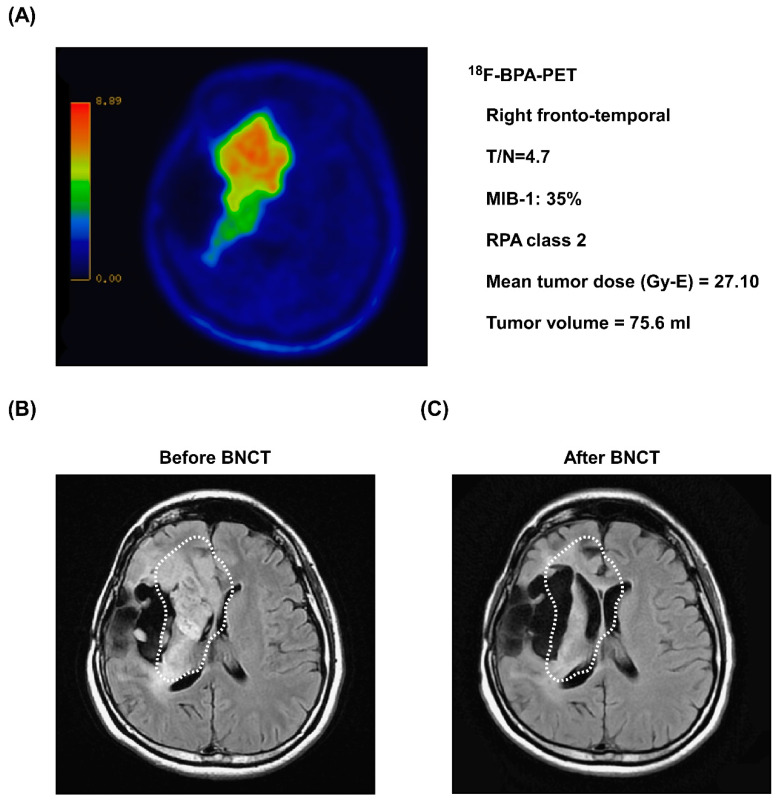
Representative images of tumor shrinkage after BNCT treatment. (A) Before BNCT treatment, ^18^F-BPA-PET revealed the location of strong tumor activity in the right fronto-temporal lobe. (B) MRI imaging of the patient before BNCT treatment. An anaplastic astrocytoma was observed in the right temporal lobe (white dashed circles). (C) Three months after BNCT treatment, a follow-up MRI image of the patient showed a significant reduction in tumor volume (white dashed circles).
